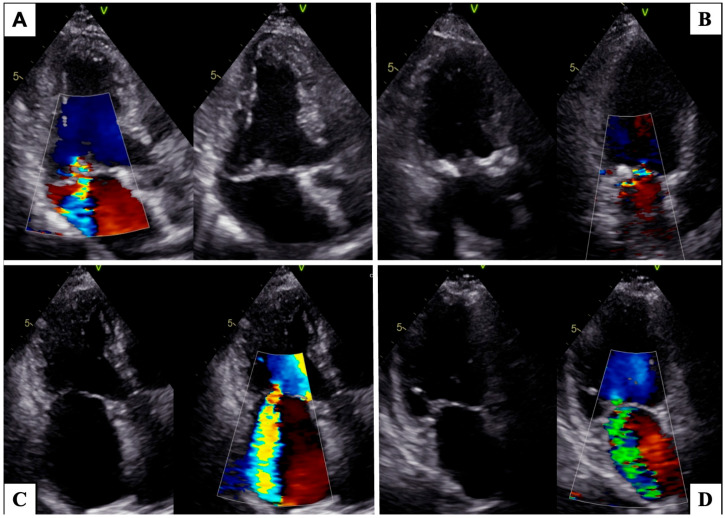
Morphological features more frequently found in female patients on bidimensional echocardiography. Barlow’s disease with multi-scallop and bileaflet prolapse (A). Extensive mitral annular calcification (B). Excessive annular dilatation in atrial functional mitral regurgitation (C). Posterior leaflet tethering in ischemic functional mitral regurgitation (D).
